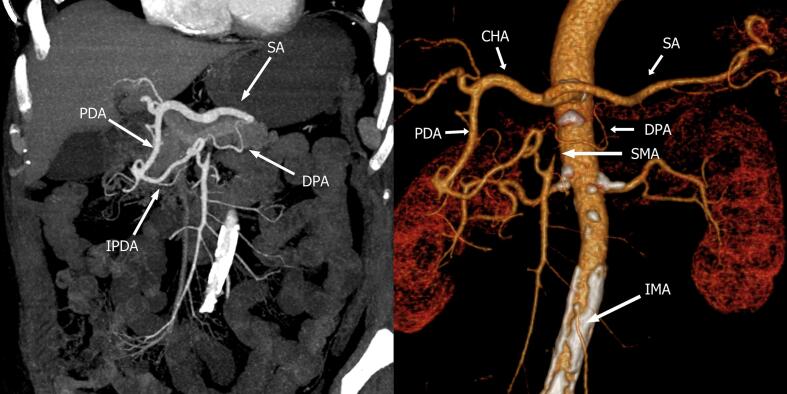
Preoperative CT-reconstruction of arterial blood supply. Shows collateral circulation from celiac axis to SMA through pancreaticoduodenal arcade (PDA) and dorsal pancreatic artery (DPA).
